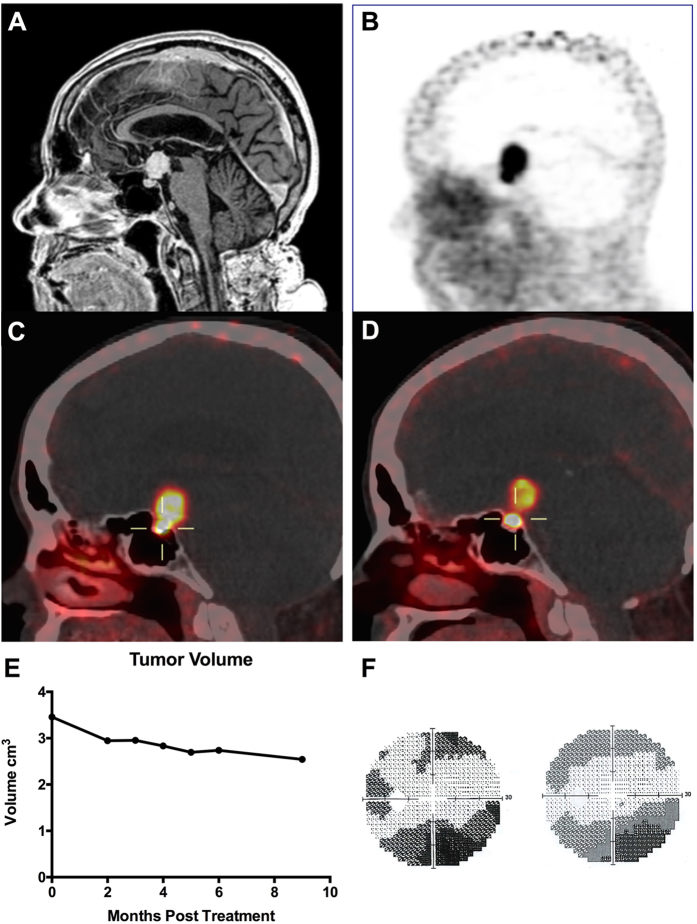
Symptomatic, surgically unresectable VHL-HB treated with octreotide. (A) T1 magnetic resonance image with contrast of 64 year-old female patient with germline *VHL* missense mutation presents with a 3.45 cm^3^ suprasellar HB. (B) ^68^GA-DOTATATE PET/CT imaging of suprasellar HB showing avid ^68^GA-DOTATATE uptake. (C) ^68^Ga-DOTATATE-PET imaging at presentation and 6 months after octreotide treatment (D). The pituitary stalk HB exhibits diminished uptake of 12.0 SUV compared to 22.6 SUV in initial study. Central photopenia also noted, indicative of necrotic process. (E) Tumor volume was measured using Osirix® software on FLAIR images. Seven images were analyzed, with the first image acquired just prior to initiation of Octreotide treatment. Measurements in cm^3^. (F) Visual field analysis of patient’s left visual field prior to starting octreotide treatment (left) and 6 months after beginning treatment.
